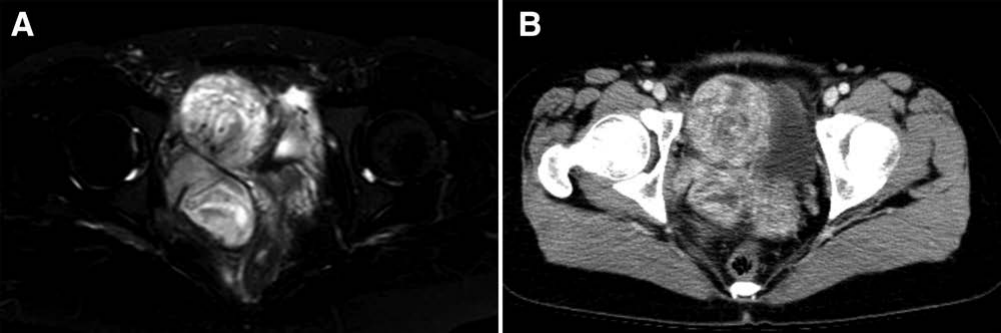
Imaging findings of aggressive angiomyxoma. The characteristic “swirling sign” within the mass was revealed by enhanced computed tomography scans (A) and magnetic resonance imaging (B).
